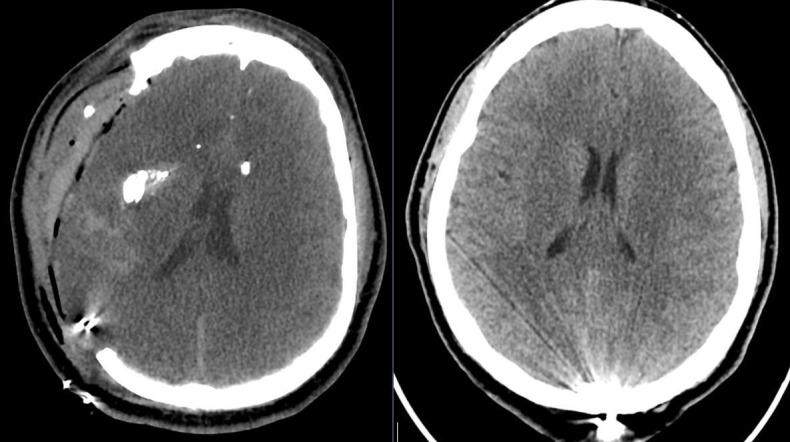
Computed tomography (CT) head revealing improved subfalcine herniation.
